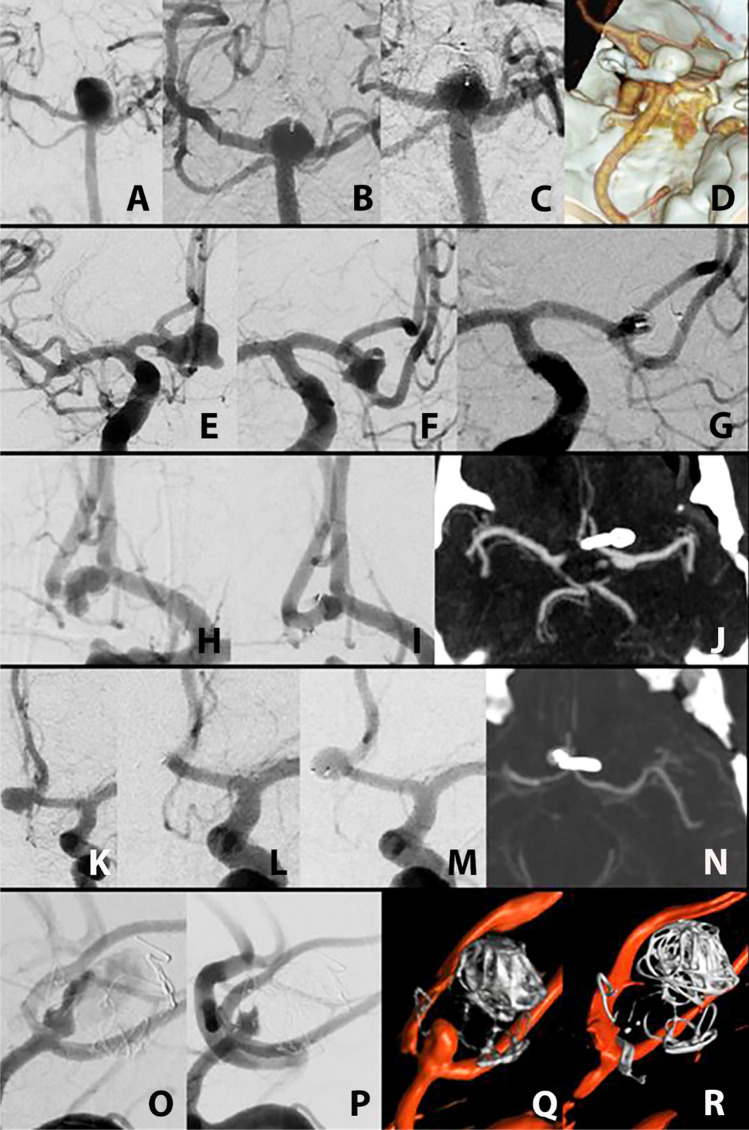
Course from the initial angiogram to the final outcome in every patient. A–D (patient 1): A shows the untreated basilar tip aneurysm, B a neck remnant equivalent to RROC II or WOS C after WEB, C the deformation of the device and progression to WOS D, and D the result after clipping. E–G (patient 2): E shows the AcomA aneurysm on initial DSA, F the radiopaque marker of the WEB protruding into the neck, and G demonstrates the position of the clip and the small neck remnant. H–-J (patient 3): H demonstrates the AComA aneurysm prior to treatment, I the neck remnant after WEB, and J the clip placement on CTA. K–N (patient 4): K demonstrates the untreated AcomA aneurysm, L complete occlusion after WEB, M the deformation of the device with complete refilling of the aneurysm, and N the result after clipping. O–R (patient 5): O demonstrates the reperfusion of the large initially ruptured and coiled AComA aneurysm, P the result after placement of the WEB within the aneurysm neck, Q the reperfusion of the aneurysm neck, and R the final result after clipping on 3D angiography
